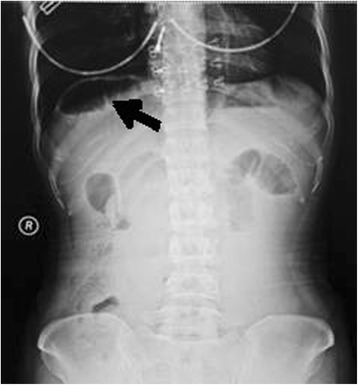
Abdominal X-ray plain film. The *black arrow* shows a gas loop of small intestine
